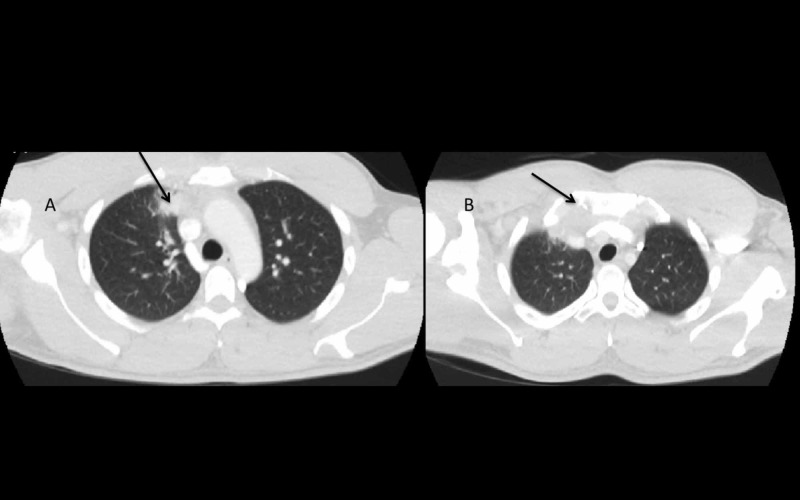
CT of the chest with contrast. A: CT chest with contrast showing focal 2.2 cm x 2.6 cm x 2.9 cm anterior-superior mediastinal mass with invasion into the right lung parenchyma (see arrow). B: CT chest with contrast showing focal anterior-superior mediastinal mass infiltrating the manubrium sterni (see arrow).
